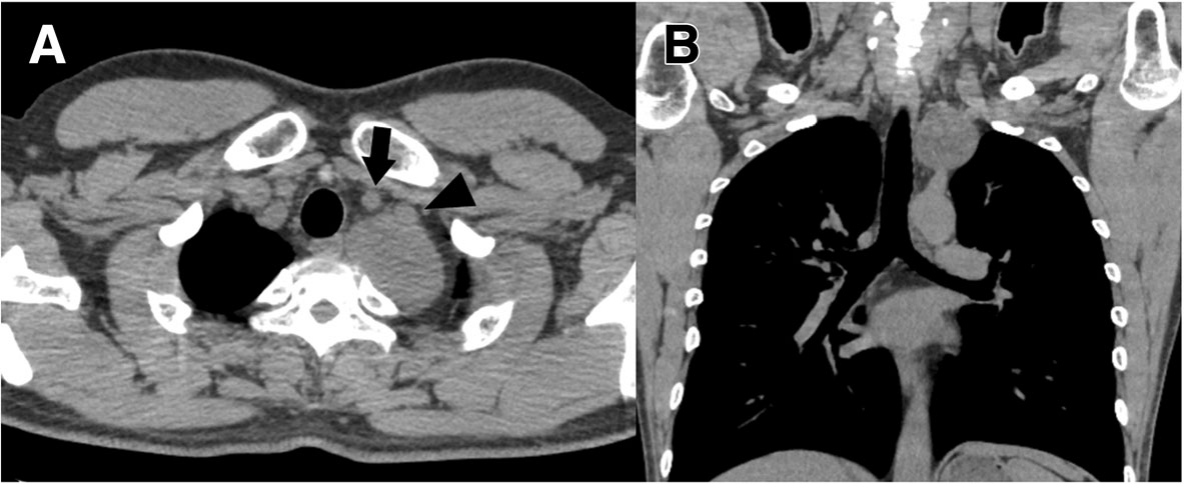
Axial (a) and coronal (b) views of the chest computed tomogram. The tumor was adjacent to the left common carotid (arrow) and left subclavian (arrowhead) arteries
